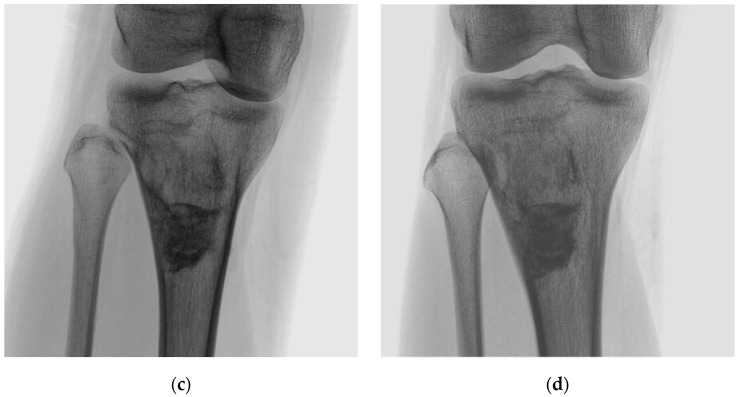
Preoperative coronal (a) and axial (b) T1-weighted MRI sequences, showing fibrous dysplasia in the right proximal tibial metaphysis (*Case 3*). Anteroposterior (AP) radiographs of right knee: (c) at 6 months follow-up, the GeneX shows partial resorption and the fenestration site is still visible on the lateral cortex; and (d) at 12 months follow-up, the GeneX continues to resorb, with progressive ossification of the defect, and the lateral cortex has healed.
